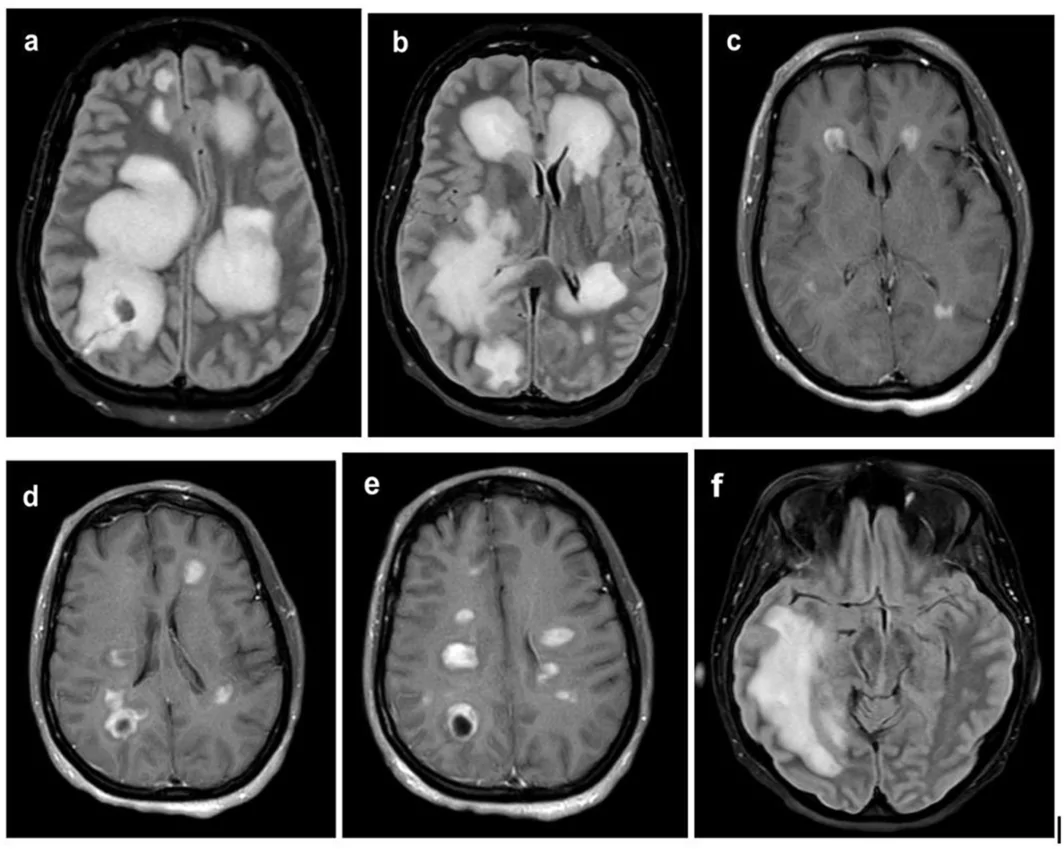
MRI brain Axial FLAIR slices obtained on day 43 of symptom onset, demonstrating significantly increased edema surrounding diffuse concentric demyelinating white matter lesions with leftward midline shift (a–c). These images were obtained after the completion of high‐dose intravenous steroids, plasma exchange, intravenous immunoglobulin, and cyclophosphamide therapies. Post‐contrast axial T1 views within the same study (d–f).
